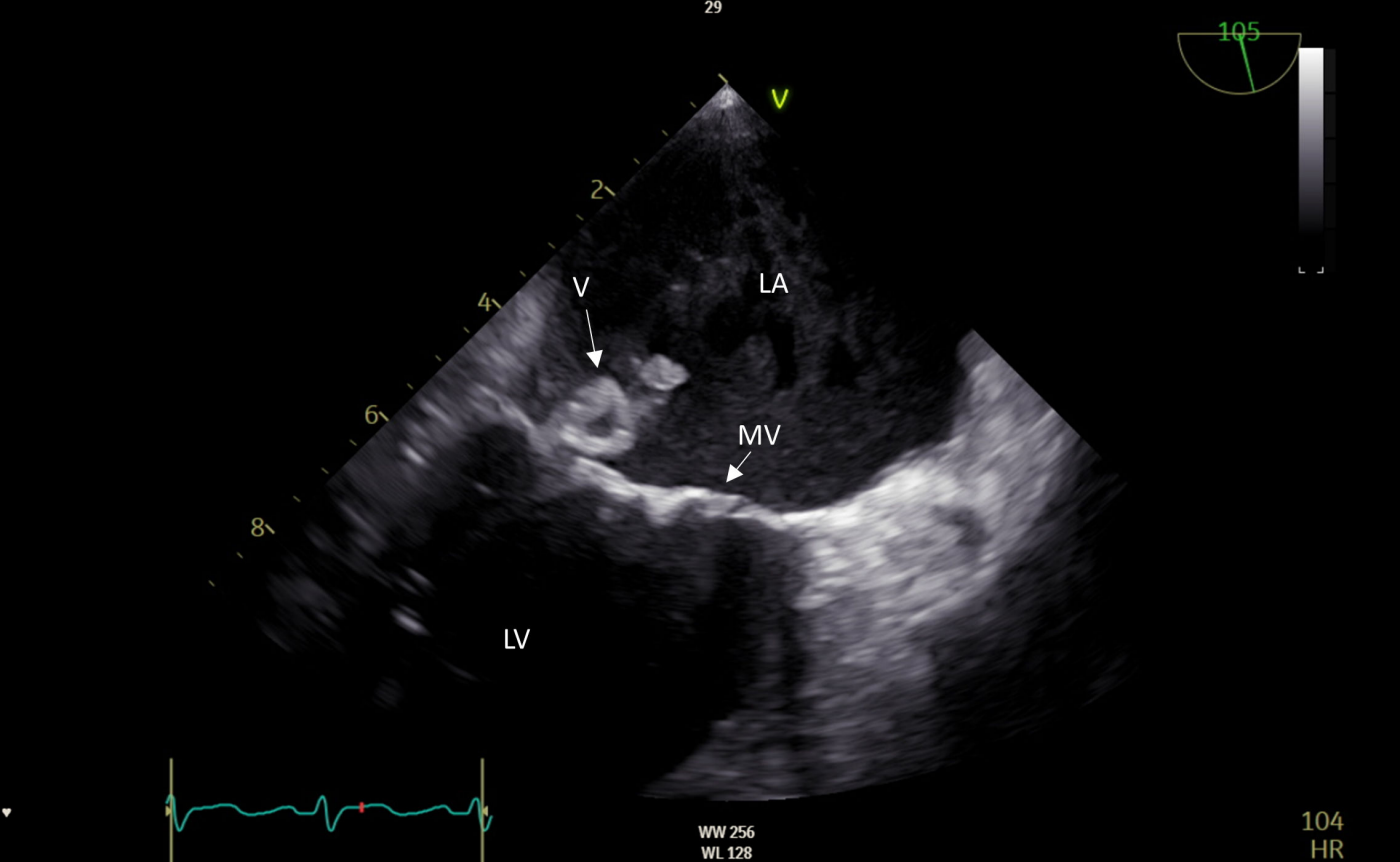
First transesophageal echocardiogram (TEE) of the patient. The TEE revealed a large vegetation of the posterior mitral leaflet. Further, in relation to the vegetation, the mitral valve was perforated, and the infection extended to the annulus of the valve, and a ruptured abscess in relation to the mitral valve was found. LA: left atrium, LV: left ventricle, MV: mitral valve, V: vegetation.
